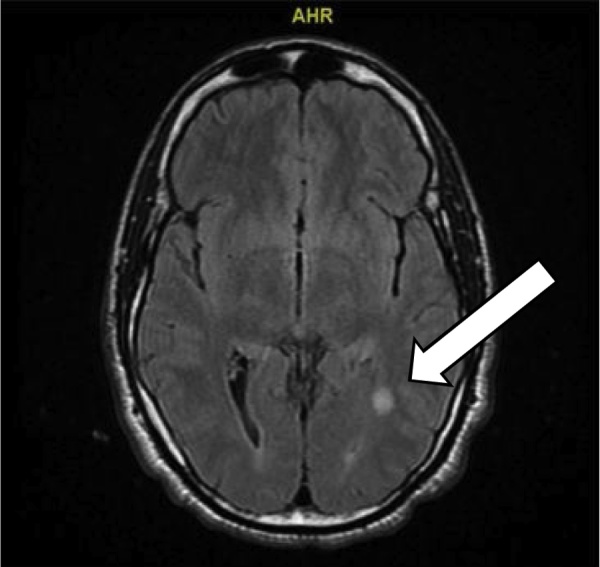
Coronal view showing the same "spot" in the left temporal- occipital white matter. This is a 10 × 8 mm T2/FLAIR white matter hyperintensity at the junction of the left temporal and occipital lobes. The lesion is isointense, does not enhance, did not restrict and does not demonstrate blooming artifact (to suggest hemorrhage).
